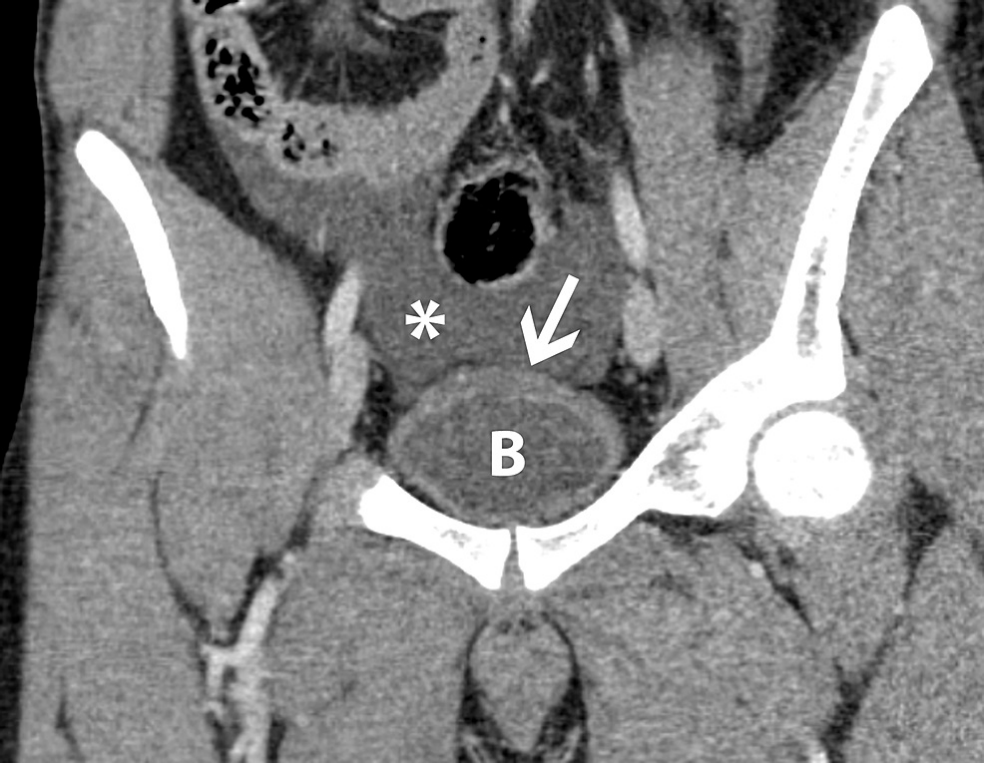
Coronal reformatted CT image shows fluid (white asterisk) in the pelvis superior to the urinary bladder (B). There is mild thickening of the superior bladder surface (white arrow).
